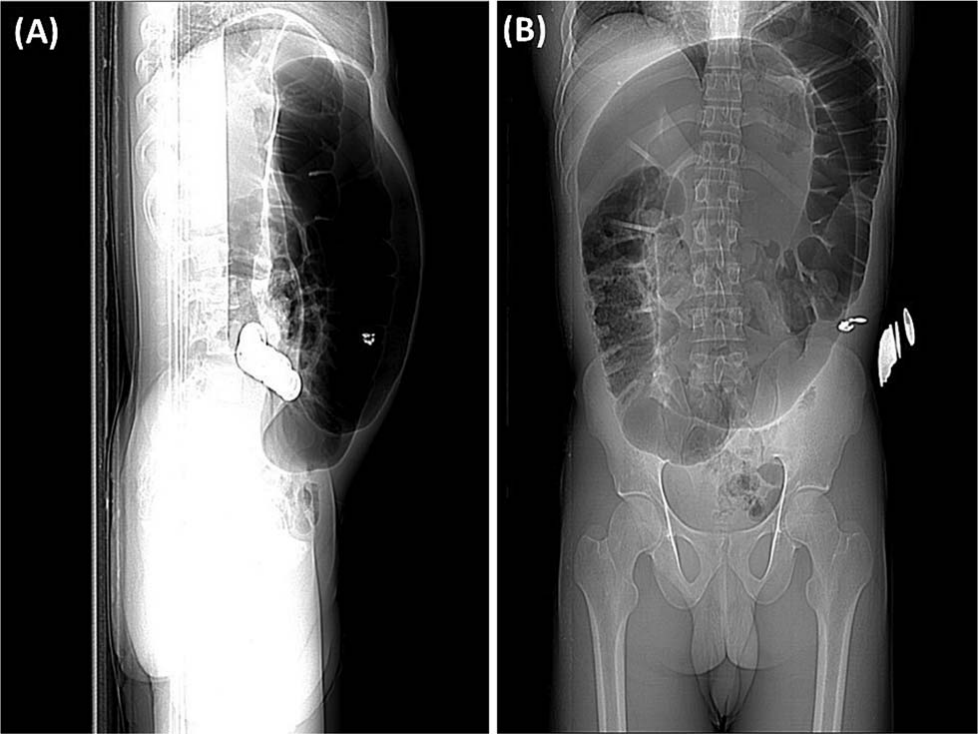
(A) The preliminary view on abdominal CT (scout view) revealed a distended sigmoid loop exhibiting an inverted U-shape, commonly recognized as the coffee bean sign. (B) The preliminary view on abdominal CT (scout view) showed dilated bowel loops.
